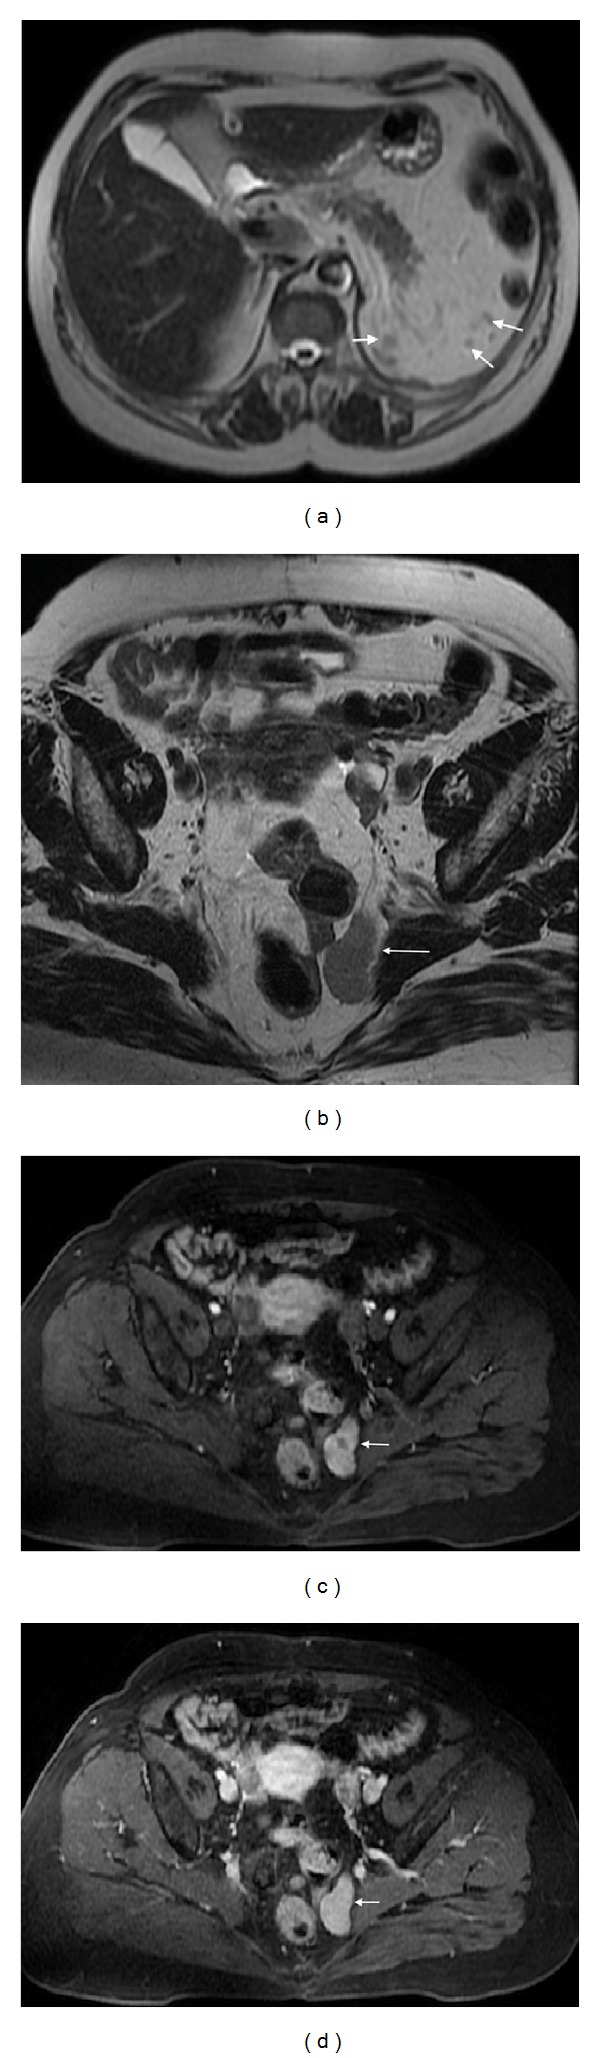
Pelvic splenosis. Axial (a) T2wi SSFSE, axial (b) T2 wi FSE and postcontrast axial T1wi 3D-GRE with fat suppression in the arterial (c) and venous (d) phase. This patient with history of splenectomy following a car accident underwent pelvic MRI to clarify pelvic masses depicted in previous CT. There are multiple nodular masses in the left hypochondrium (arrows, (a)) consistent with splenosis. There are also multiple well-defined nodules in the pelvis demonstrating high signal intensity on T2wi (arrow, (b)), with heterogeneous enhancement immediately after contrast material administration (c), becoming homogeneous in the venous (d) phase, consistent with splenosis.
